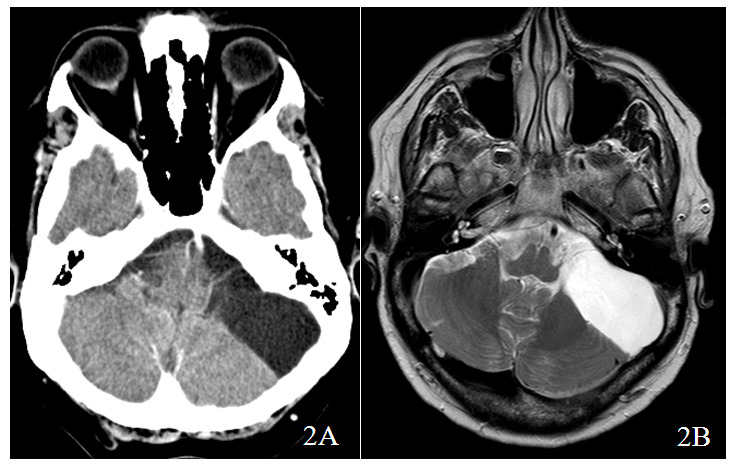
Head computed tomography (CT) scan showed the pre-existing cystic lesion in the left cerebellopontine angle with a slight right brainstem deviation, without associated edema (
